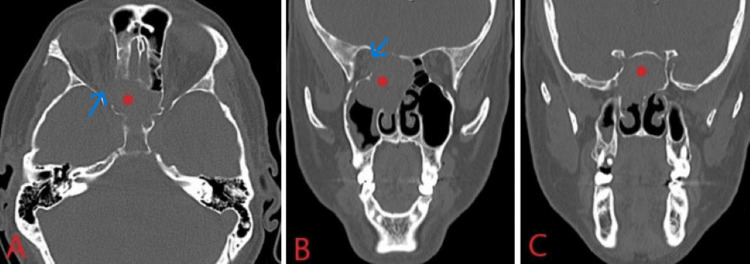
CT maxillofacial without contrast (A), coronal (B), and axial planes (C) in a bone window, showing 4.5 cm x 4.1 cm x 4.0 cm soft tissue mass (red dot) centered in the right ethmoid air cells involving the right sphenopalatine foramen, right olfactory recess, bilateral sphenoid sinuses, and right maxillary sinus. Noted bony destruction of the medial orbital apex and abutment of the right optic nerve (blue arrow), thinning of the right planum sphenoidale and posterior medial right orbital wall, and enlargement of the right maxillary osteomeatal complex (OMC) and posterior fontanelle.
